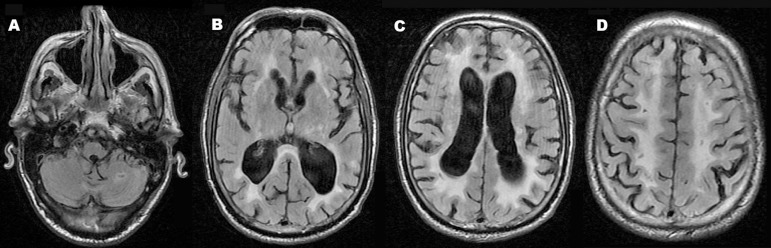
Axial FLAIR images. Note a hyperintense lesion with a hypointense
